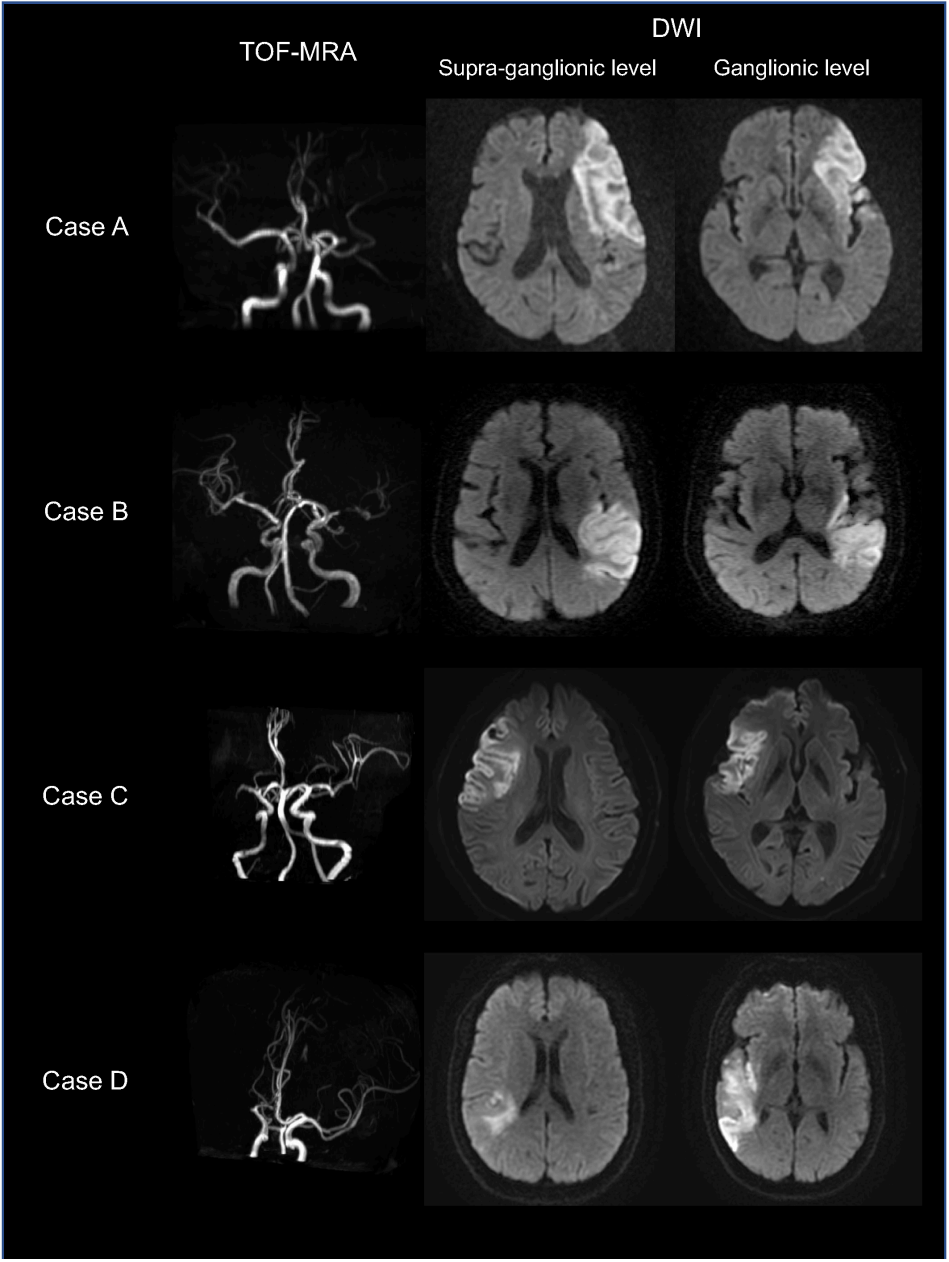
Representative cases of patients with different ASPECTS subregions involvement and clinical outcomes. Case A: A 73-year-old man with left MCA occlusion on TOF-MRA. The baseline NIHSS score was 12. Posttreatment MR-DWI showed the infarct lesion involving the left insula, M1, M4, and M5 regions with an ASPECTS of 6. This patient received intravenous thrombolysis therapy yet suffered moderately severe disability with a 3-month mRS score of 4. Case B: A 73-year-old woman with left MCA severe stenosis and baseline NIHSS score of 9. The infarct lesion affected the left insula, M3, M5, and M6 (ASPECTS score = 6). This patient received medical therapy and presented slight poststroke disability with a 3-month mRS score of 2. Case C: A 69-year-old man with right MCA occlusion and baseline NIHSS score of 8. The infarct lesion involved the right insula, M1, M2, M4, and M5 (ASPECTS score = 5). This patient presented slight poststroke disability with an mRS score of 2 at 3 months. Case D: A 53-year-old man with right MCA occlusion and baseline NIHSS score of 4. Posttreatment DWI showed the infarct lesion involving the right insula, M2, M3, and M6 (ASPECTS score = 6). This patient presented moderate disability with a 3-month mRS score of 3. MCA: middle cerebral artery; TOF-MRA: time-of-flight MR angiography; NIHSS: National Institute of Health Stroke Scale; mRS: modified Rankin Scale; ASPECTS: Alberta Stroke Program Early CT Score; MR: magnetic resonance; DWI: diffusion-weighted imaging.
